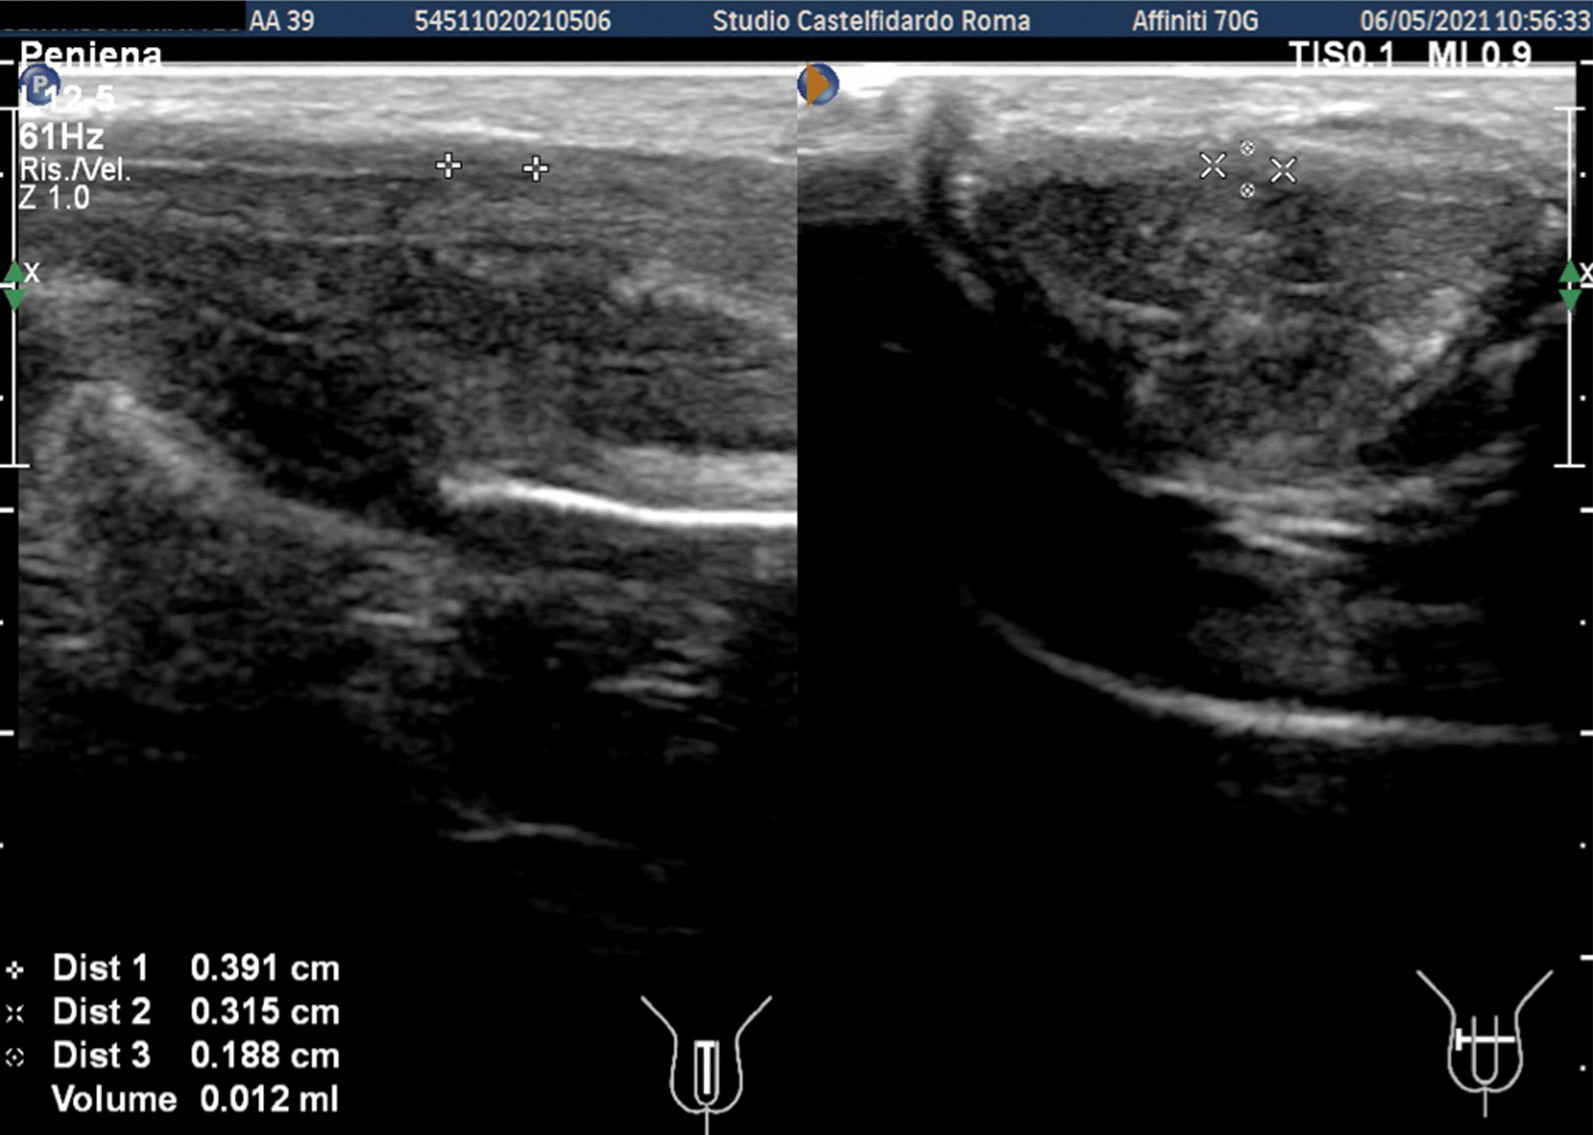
Penile ultrasound after the third treatment cycle (longitudinal and transverse scan)
